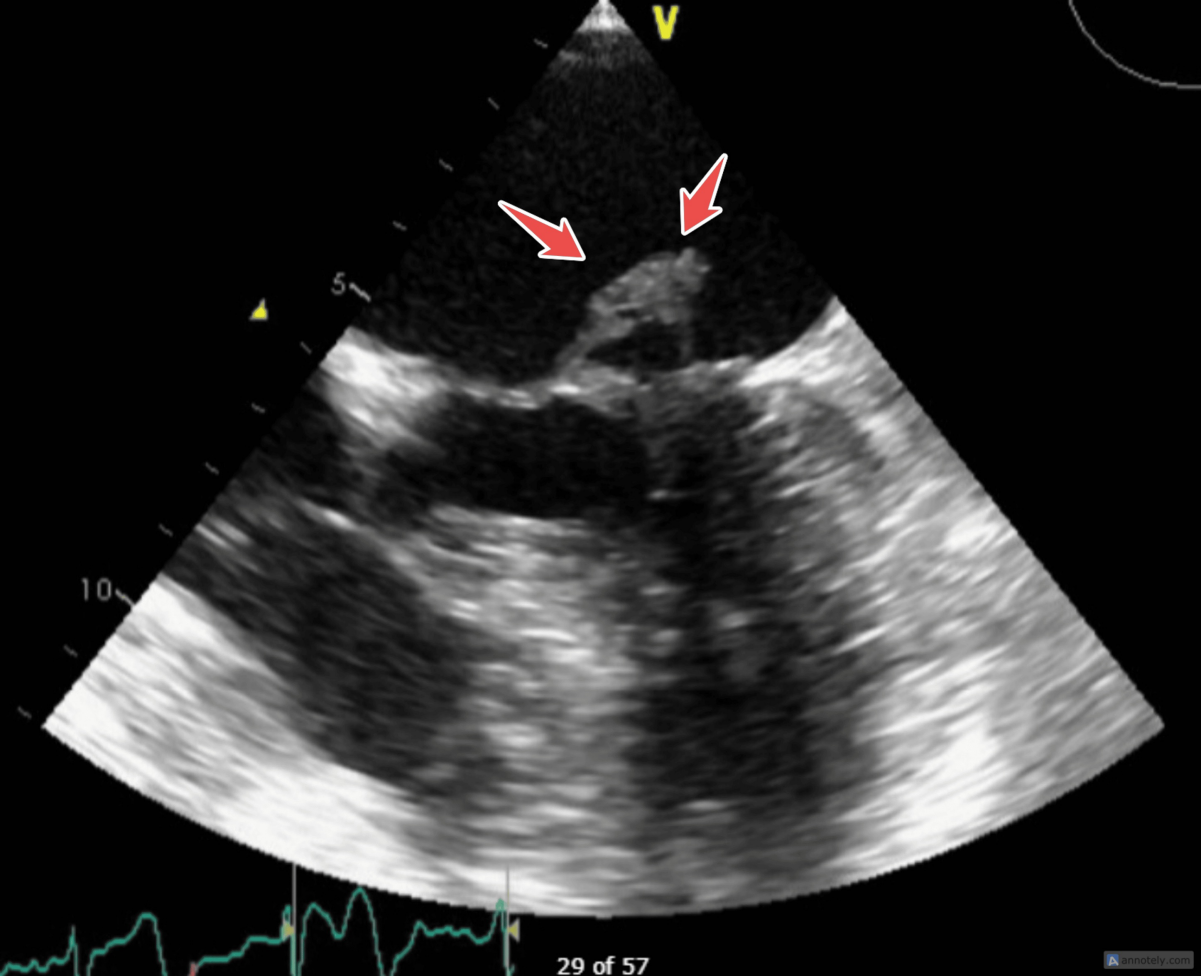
TEE showing vegetation highlighted by arrows. TEE: Transesophageal echocardiogram.
